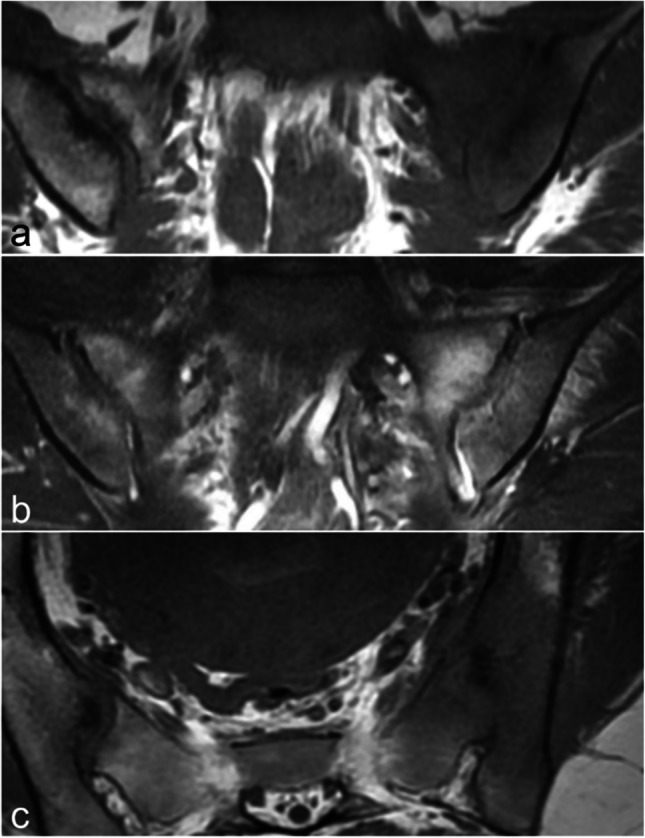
A 21-year-old woman, 9 days post-partum. Coronal oblique T1- (a) and T2 (b)-weighted images, axial oblique T1-weighted image (c). On the right side, mechanical changes related to pregnancy are observed, with subchondral sclerosis surrounded by mild BME; on the left side, there is extensive and pronounced subchondral BME with significant infiltration of the adjacent soft tissues, indicative of infectious sacroiliitis
